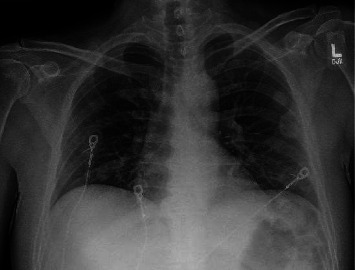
Chest X-ray showing two nodular densities measuring 2.7 and 1.6 cm in the left lung, overlying the fifth and sixth ribs, and scapula.
